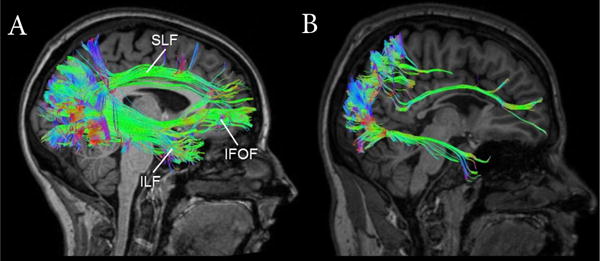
Diffusion based MRI reveals differences in white matter connectivity White matter tractography reconstruction in a normally-sighted control subject (A) compared to an age matched individual with CVI (B). In the control subject, the inferior longitudinal fasciculus (ILF), superior longitudinal fasciculus (SLF) and inferior fronto-occipital fasciculus (IFOF) are all evident. In contrast, marked reductions in each of these fasciculi is evident. Color scheme represents the direction of the white matter fibers (see Figure 1B).
